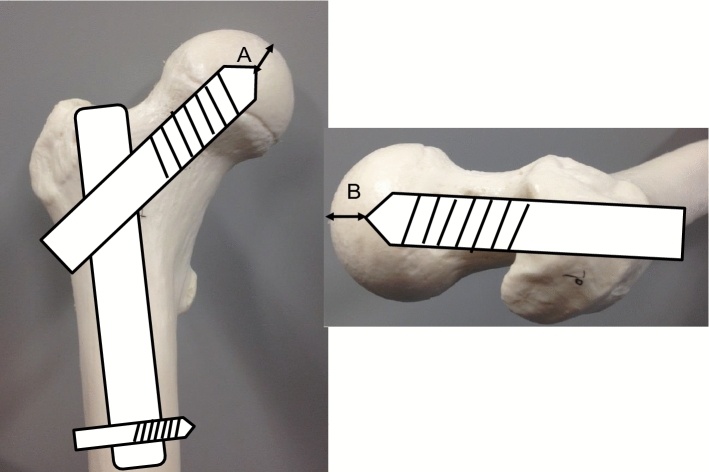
Tip-apex distance (TAD). TAD is the sum of the distance (A + B) from the tip of the lag screw to the apex of the femoral head on (A) an anteroposterior radiograph and (B) a lateral radiograph. It should be < 25 mm to prevent cut-out of the lag screw.
